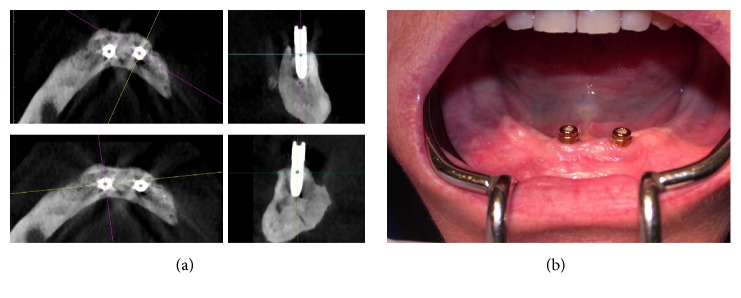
(a) Follow-up after surgery. CBCT images 12 months after osteomyelitis. (b) Intraoral photography showing the two dental implants without inflammation 12 months after osteomyelitis.
